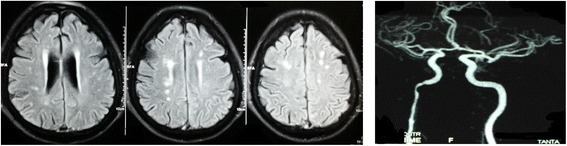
A case of bilateral internal border zone infarction with CT angiography showing severe stenosis in the cavernous and petrous portion of right internal carotid artery
